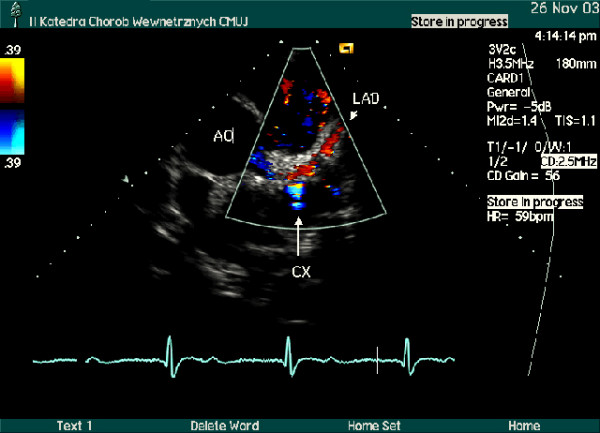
Parasternal short axis view, color Doppler examination at the heart base level. Left main, left anterior descending (LAD) and origin of left circumflex (Cx) coronary arteries are seen. Blood flow within the left main coronary artery and proximal LAD is depicted in red, while in the Cx – in blue.
